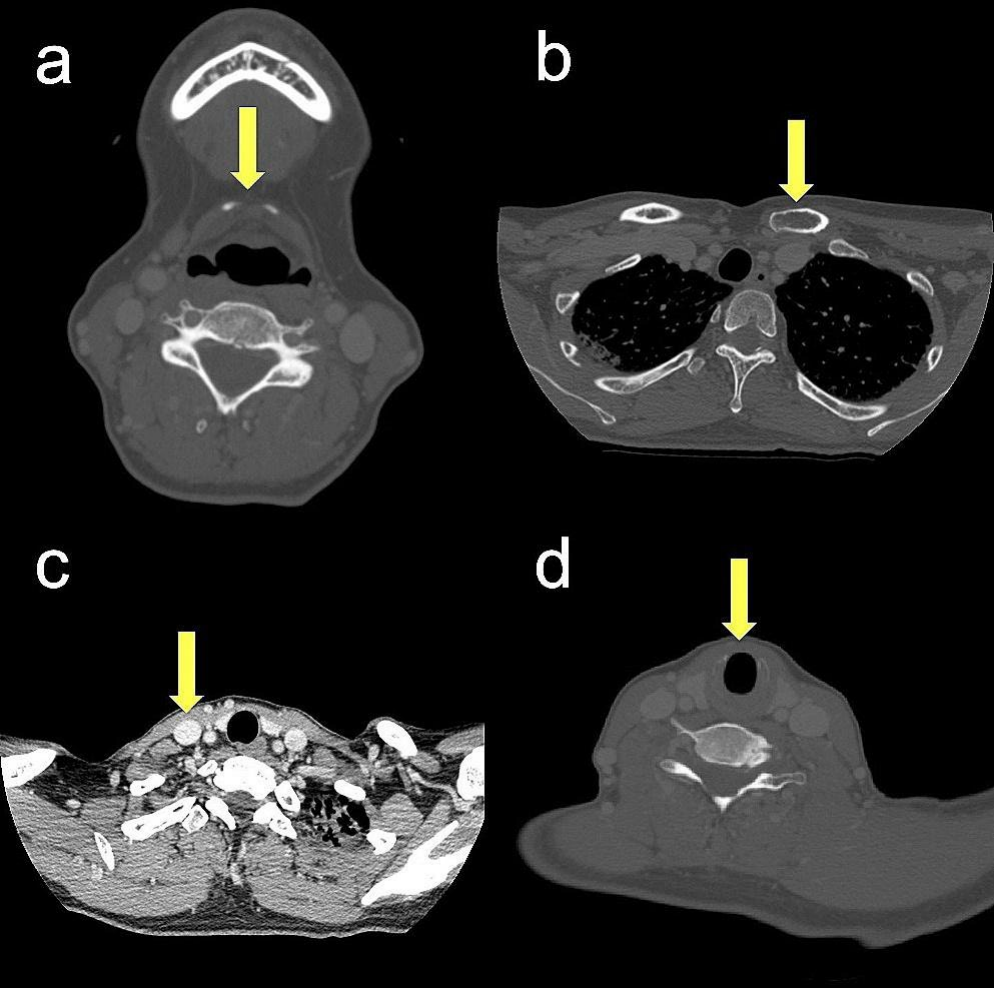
The points of measurement of anatomical structures in the slice of contrast-enhanced CT (CE-CT) images. (a) Central inferior border of hyoid bone (HB). (b) Superior border of sternal end of clavicle (CL). (c) Intersection of omohyoid muscle and internal jugular vein (OM-IJ). (d) Inferior border of cricoid cartilage (CC). The OM-IJ was identified using CE-CT images with soft tissue windows, and the other three anatomical structures (HB, CL, and CC) were identified with the bone windows
